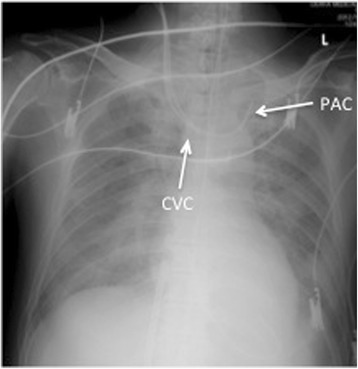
Chest X-ray reveals misplacement of the central venous catheter (*CVC*) and pulmonary artery catheter (*PAC*) in the innominate vein. *L* left
